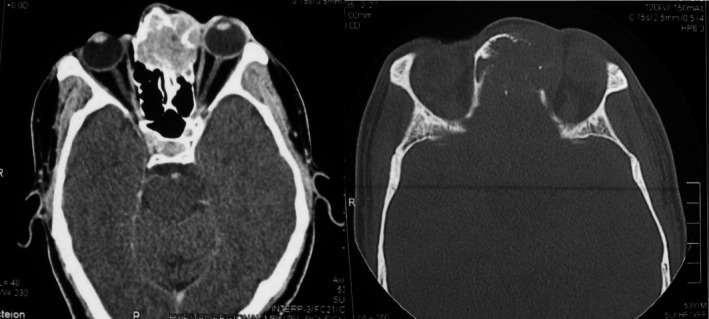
CT scan of the facial mass in axial section ethmoido‐frontal tissue process with heterogeneous enhancement following contrast injection, showing bone lysis of the inner wall of the left orbit.
